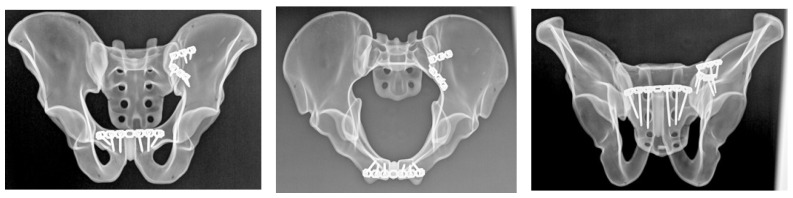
Anteroposterior, inlet, and outlet radiographs demonstrating anterior sacroiliac plate fixation of the posterior pelvic ring in the Tile C1.2 fracture model.
